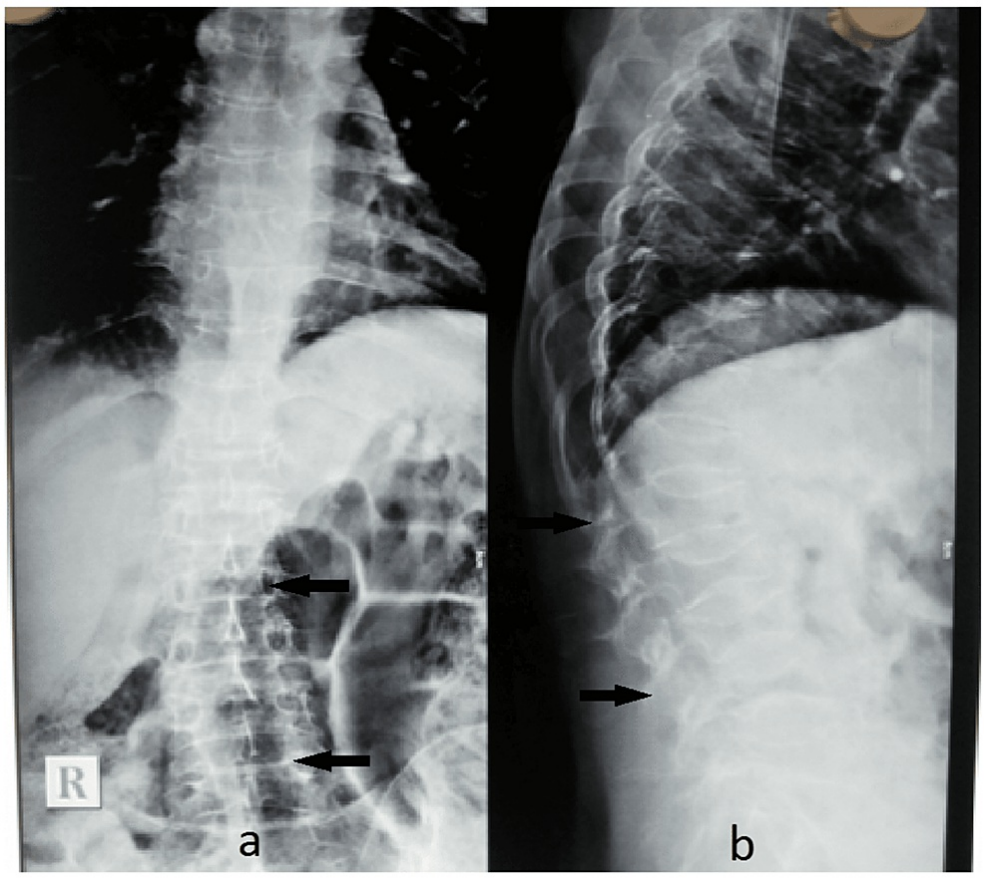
Plain X-ray of the lumbosacral spine a) Anteroposterior view; b) lateral view. The arrows indicate severe osteopenia with wedging of L1-L4 vertebrae
